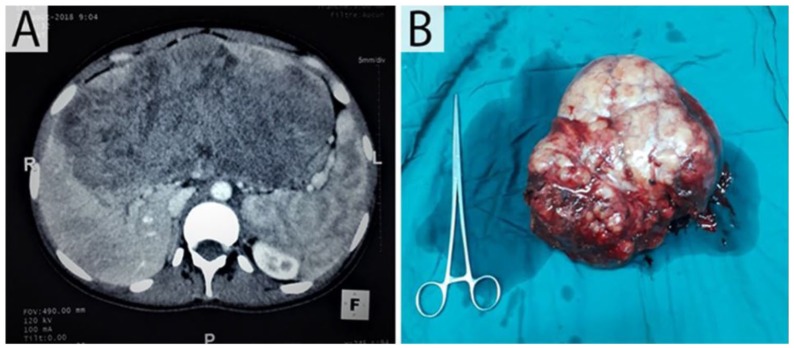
(A) Contrast-enhanced computed tomography images showed a solitary focal
